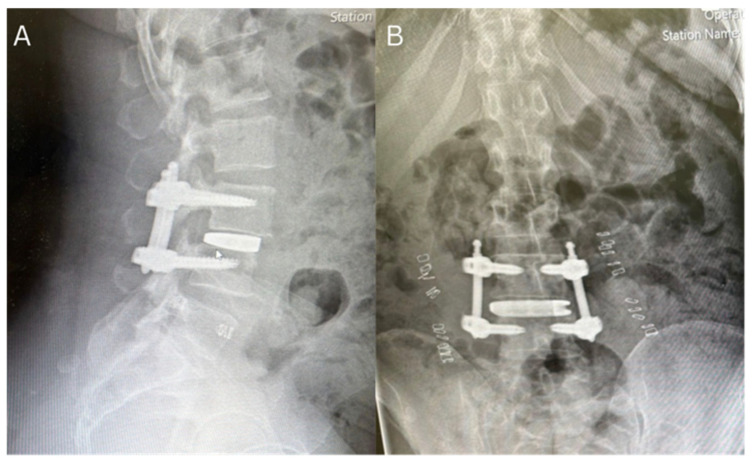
Representative post-operative plain radiographs following robotic-assisted spinal instrumentation. (A) Sagittal Xray of lumbar construct demonstrating screw placement and alignment. (B) Lumbar construct following instrumentation, confirming overall construct positioning.
